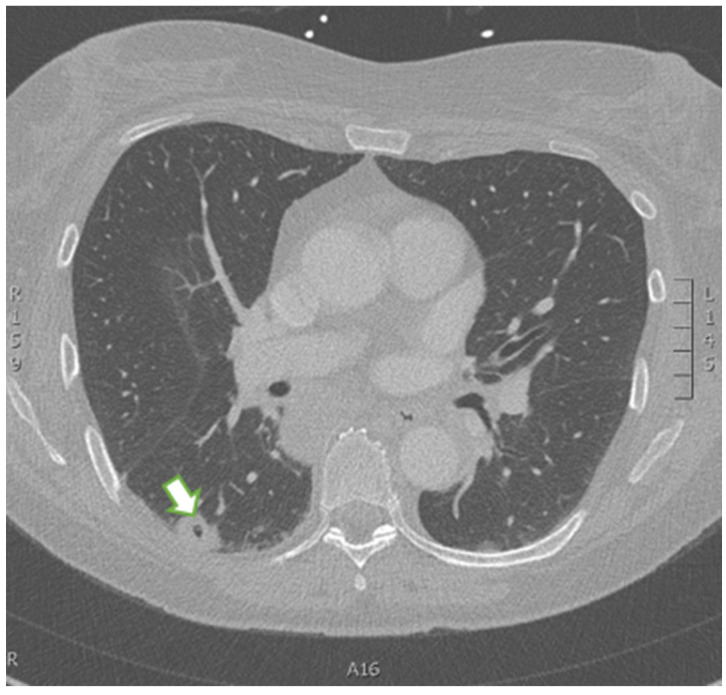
CT of the chest. The right lower lobe reveals evidence of a cavitary lesion (white arrow).
